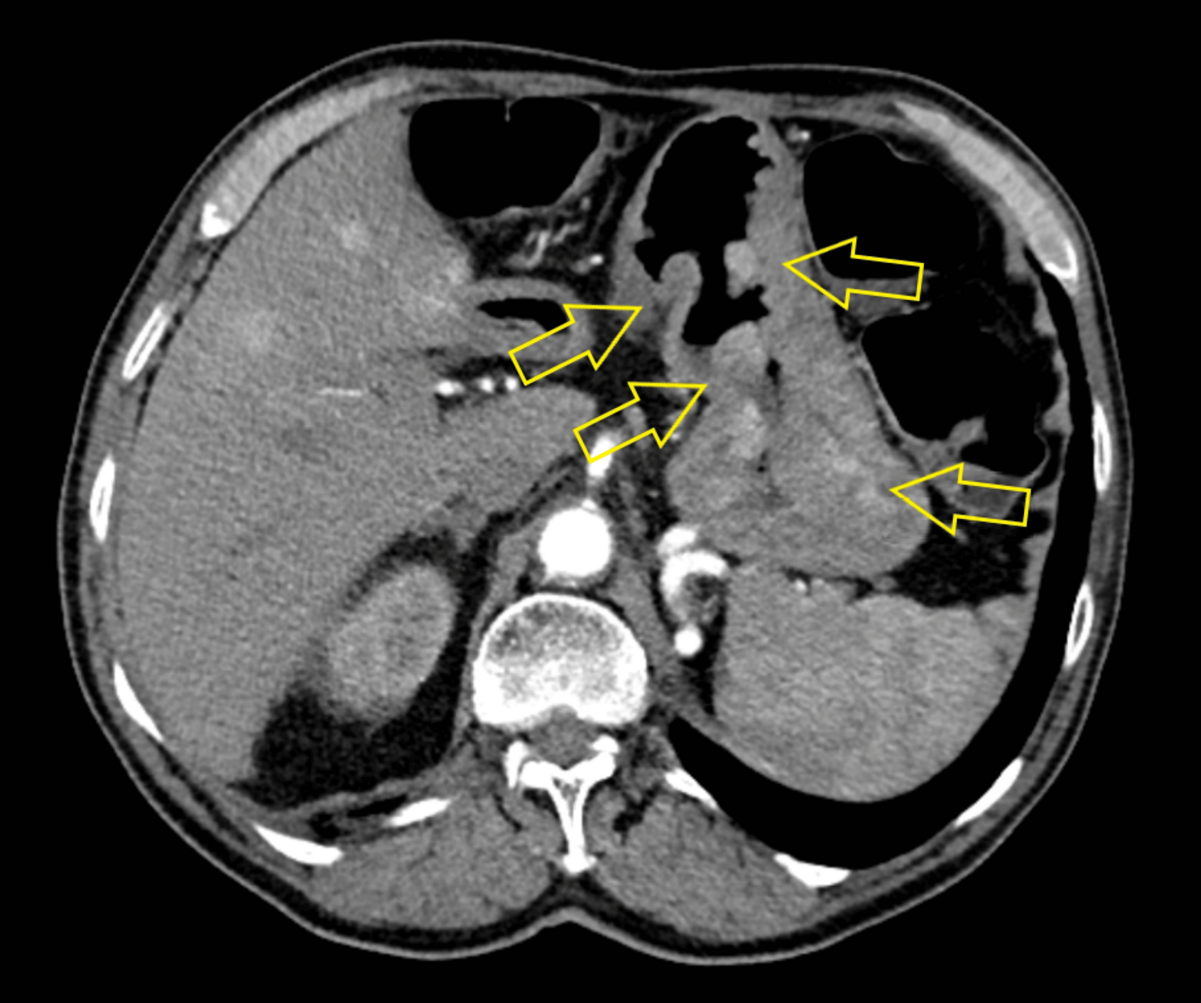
Axial contrast-enhanced CT image showing multiple nodular lesions in the gastric wall (yellow arrows).
